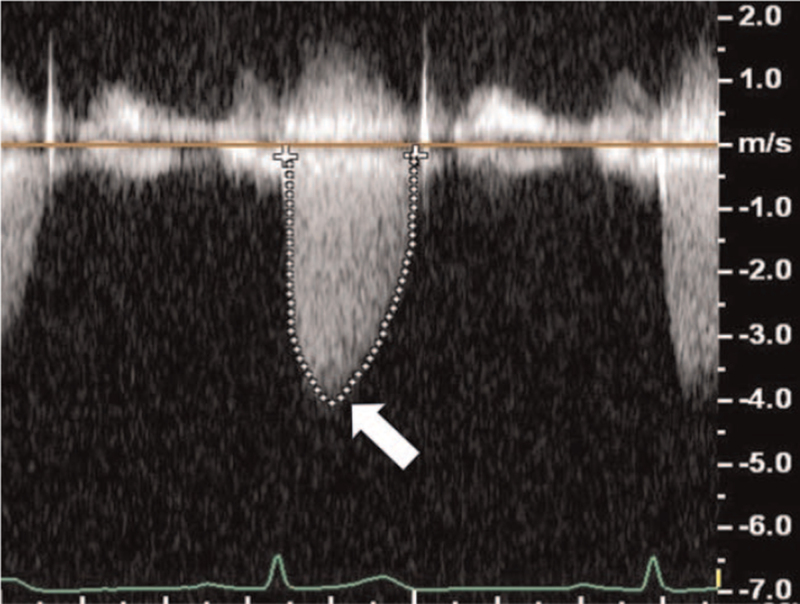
Doppler and tracing of the velocity curve of severe aortic stenosis jet. Doppler and tracing of the velocity curve of severe aortic stenosis jet showing a maximum blood flow velocity of 4.05 m/s (white arrow) and mean pressure gradient of 42 mm Hg.
